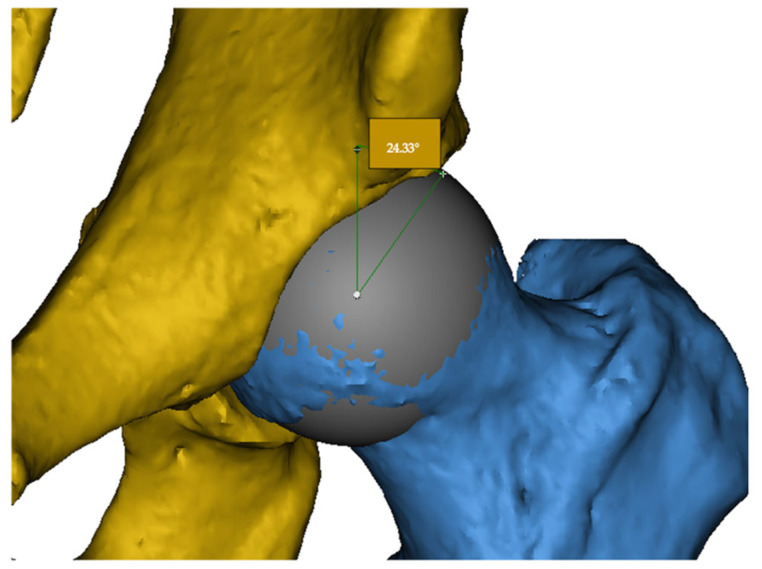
Measurement of the lateral center-edge angle (LCEA) on a 3D CT reconstruction. The acetabulum is shown in yellow and the femoral head in blue. The example demonstrates an LCEA of 24.33°.
